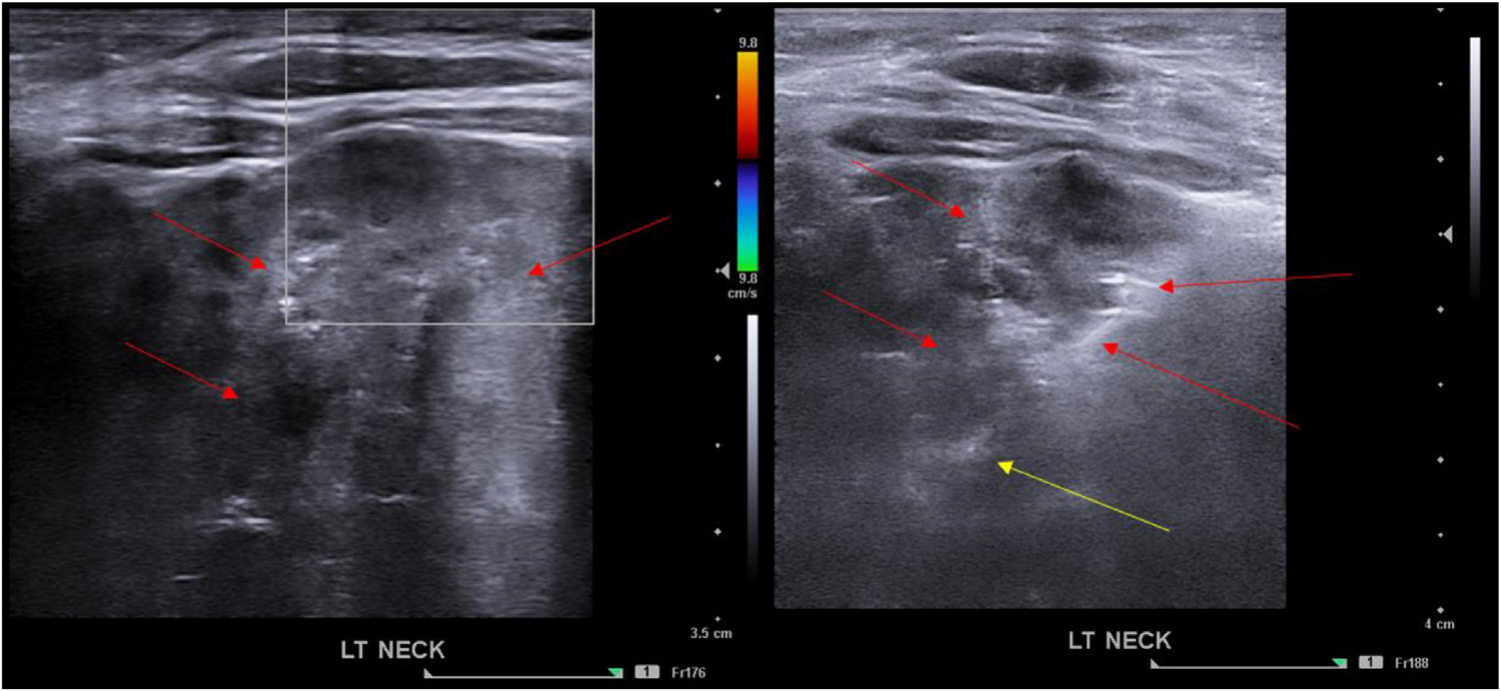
Ultrasound guided core needle (yellow arrow) biopsy from the left anterior neck approach of the heterogeneous mediastinal mass. (Color version of figure is available online.)
